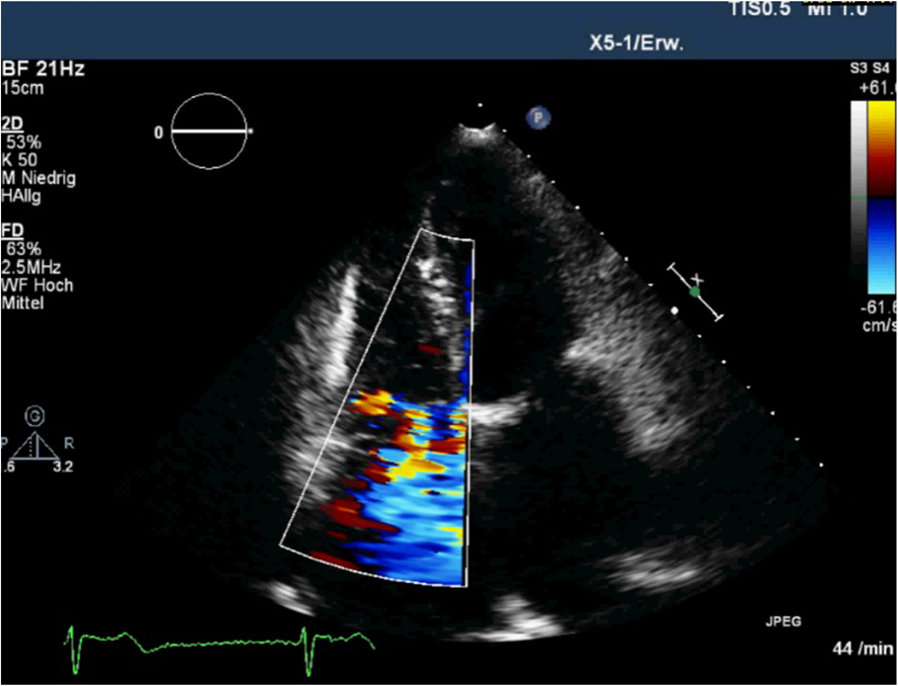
Two-dimensional color-flow Doppler echocardiogram shows the Gerbode defect as well as the low tricuspid regurgitation (arrows) in apical 4-chamber view.
